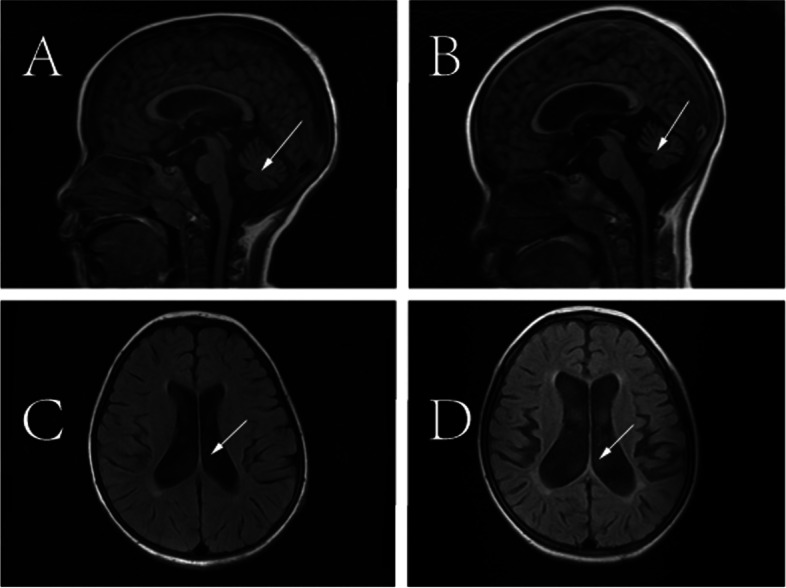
Comparison of MRI image between the first admission and recent time. A and C MRI on the first admission of the patient. B and D Recent MRI of the patient
